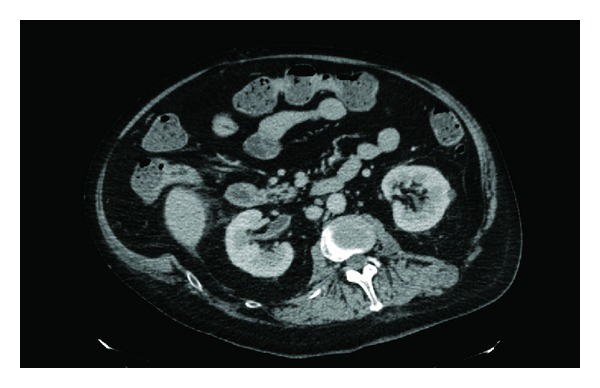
Axial section of computed tomography of abdomen and pelvis revealed inflamed uroepithelium of right renal pelvis and right ureter.
